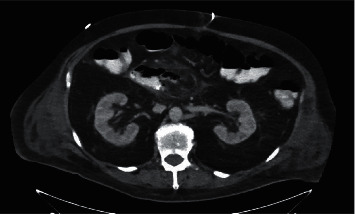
Follow-up CT scan showing resolution of anastomotic leakage.
